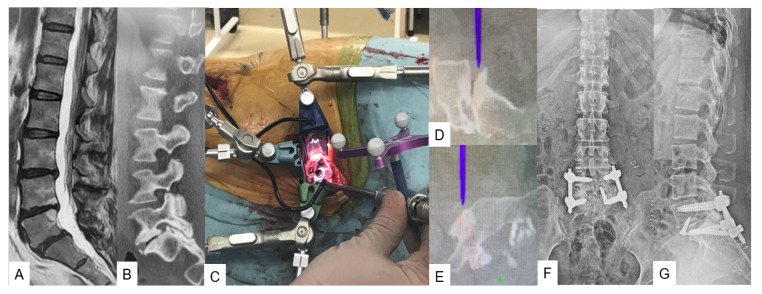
Case 4, 52-year-old female, L5 degenerative spondylolisthesis, L5/S simultaneous OLIF51 and PPS. (A) Preoperative T2 weighted MR imaging, (B) Preoperative CT, (C) Intraoperative image, (D,E) Navigation monitor, (F) Preoperative anteroposterior radiogram, (G) Postoperative lateral radiogram.
